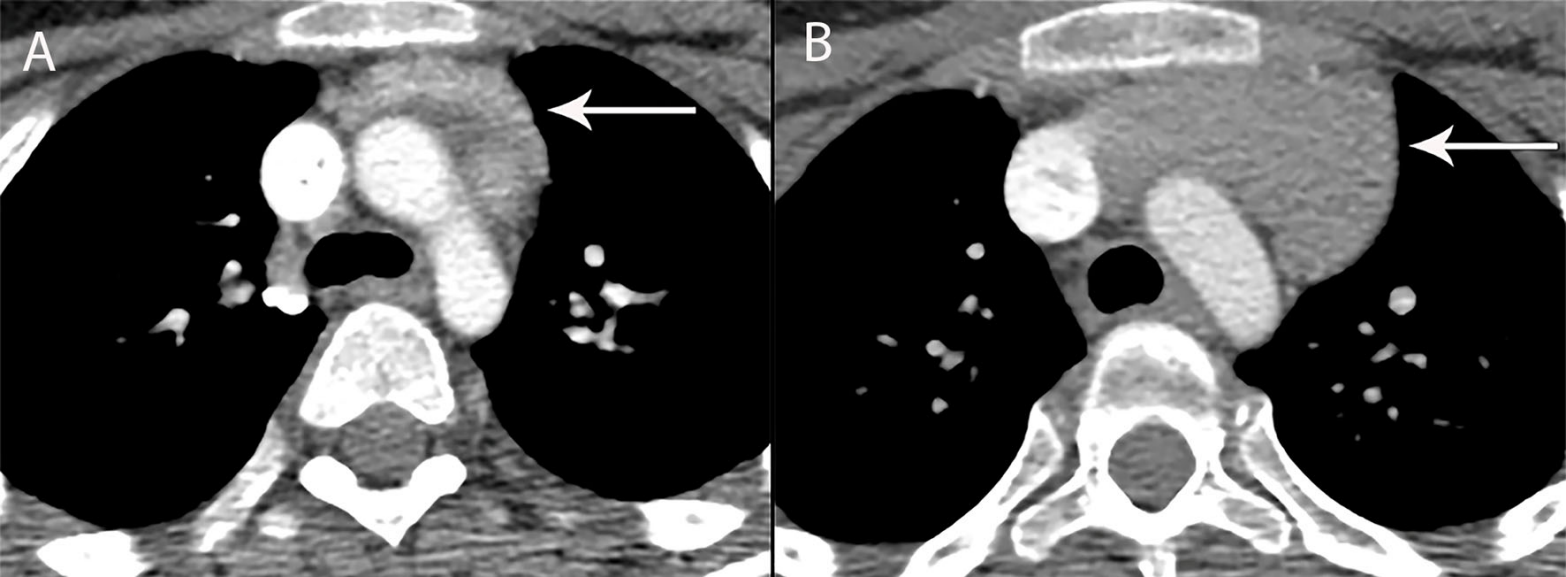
13 year old boy with chondroblastic osteosarcoma of the femur, treated with methotrexate, doxorubicin, cisplatin (A) CT shows the normal thymus (arrow) at baseline. (B) CT 4 months later shows increase in size of the thymus consistent with rebound hyperplasia (arrow). Enlargement of the thymus gland due to hyperplasia during the recovery phase from physical stress such as after chemotherapy or recovering from burns, does not displace or change the contour of vessels surrounding it. In the appropriate clinical context of thymic hyperplasia, CT is adequate for diagnosis and MRI is not needed for confirmation.
